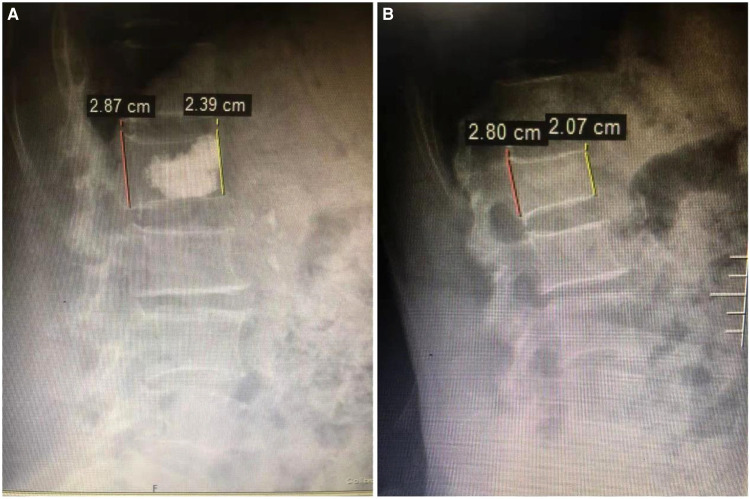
Changes in the height of the anterior vertebral body detected by X-ray before (A) and after (B) PKP treatment.
